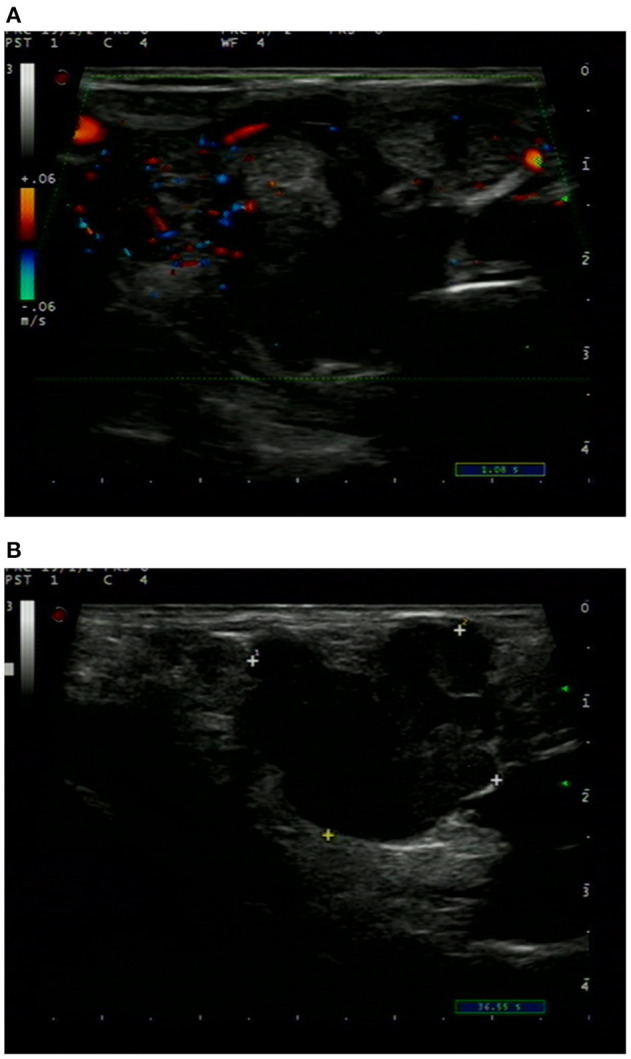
Ultrasound sonogram of the thyroid showed a non-homogenous mass with an unclear boundary, low and moderate echo and rich blood flow signal (A). Enlarged lymph nodes were found in the right neck (B).
